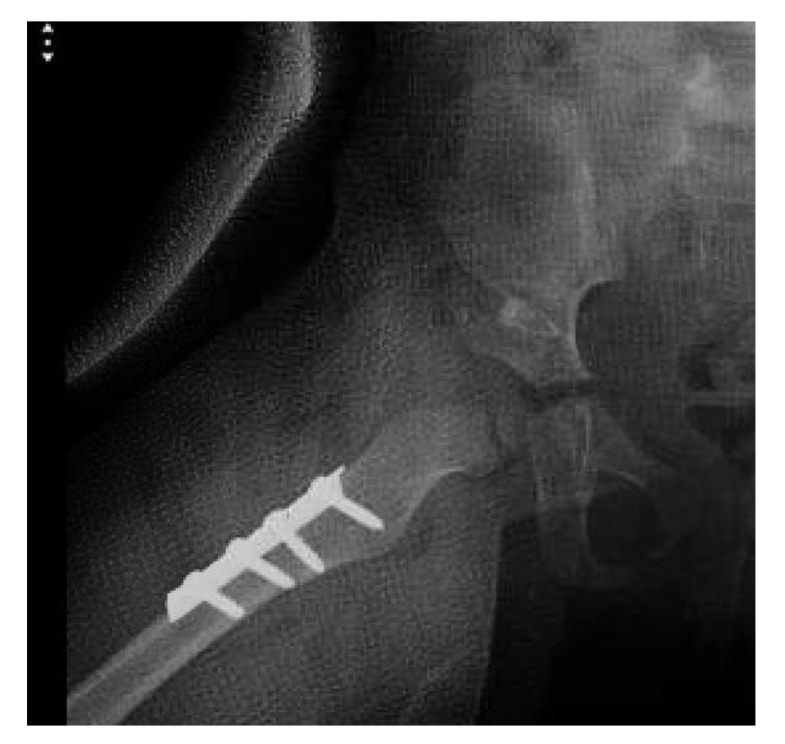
Postoperative radiograph indicating concentric reduction. Note the overlap between the ossific nucleus and the Ischium.
